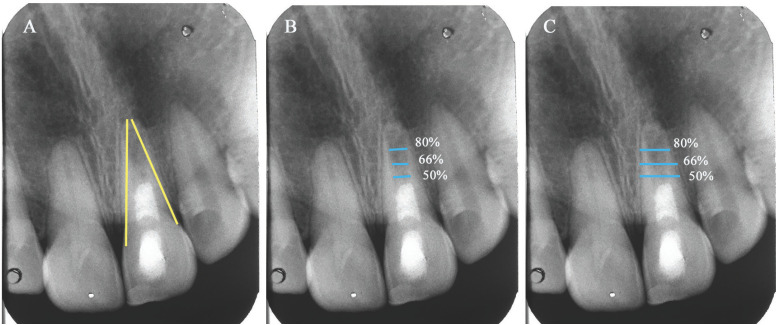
Radiographic root measurement methods, using ImageJ software. (A) Root length measurement (B) pulp space width measurement, and (C) total root width measurement. The root thickness was determined subtracting pulp space width from the total root width.
